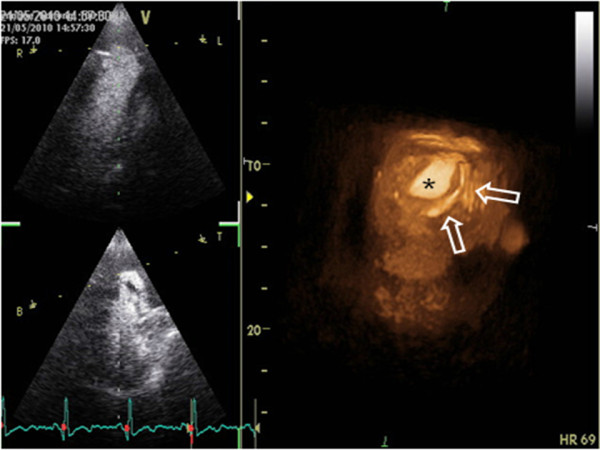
3-D echocardiography with constrast, revealing calcification of the pericardium (arrows) and echo-contrast filling the pseudoaneurysm neck (*).
